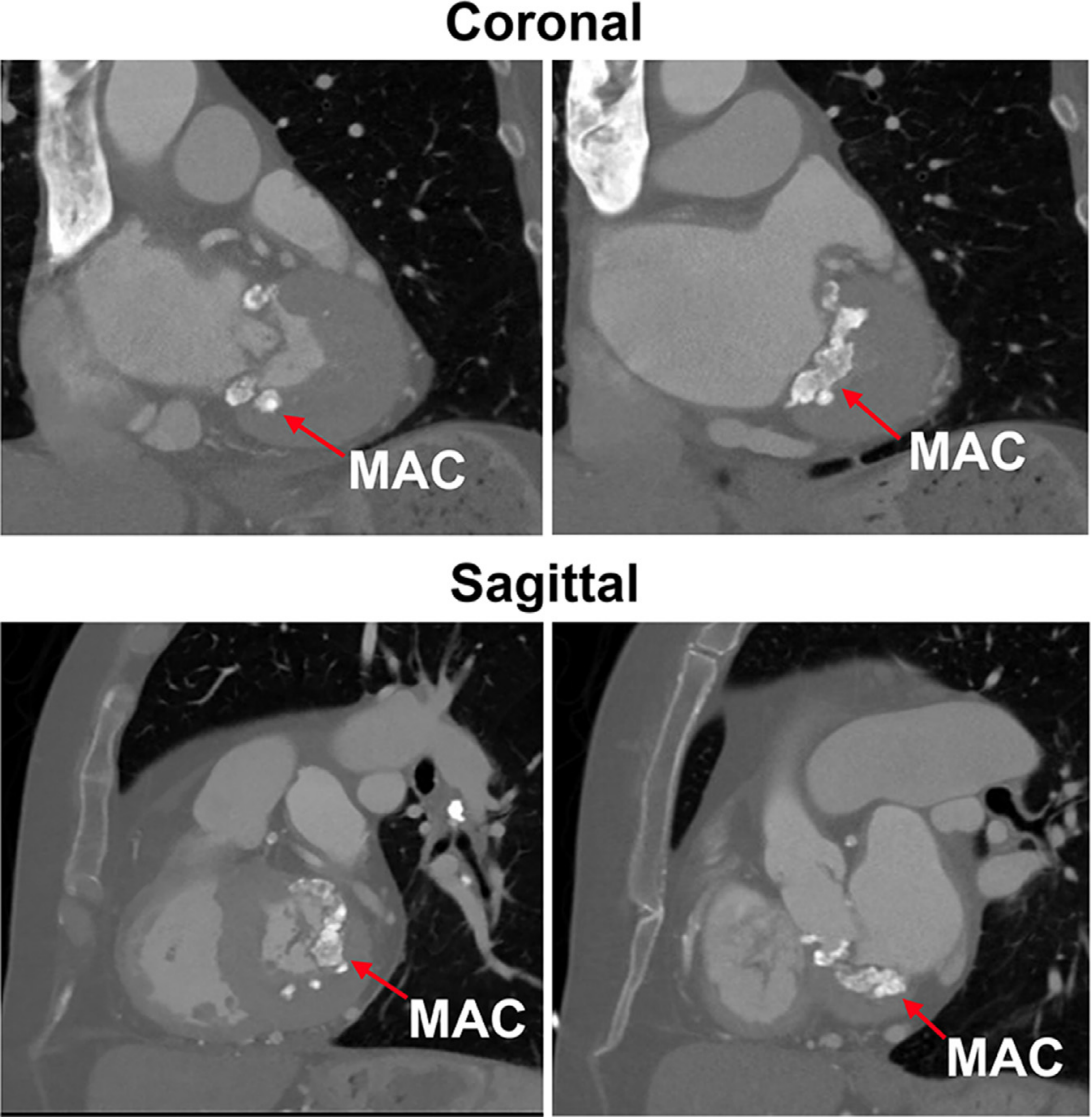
MAC shown on coronal and sagittal section on computed tomography. *MAC*, Mitral annular calcification.
